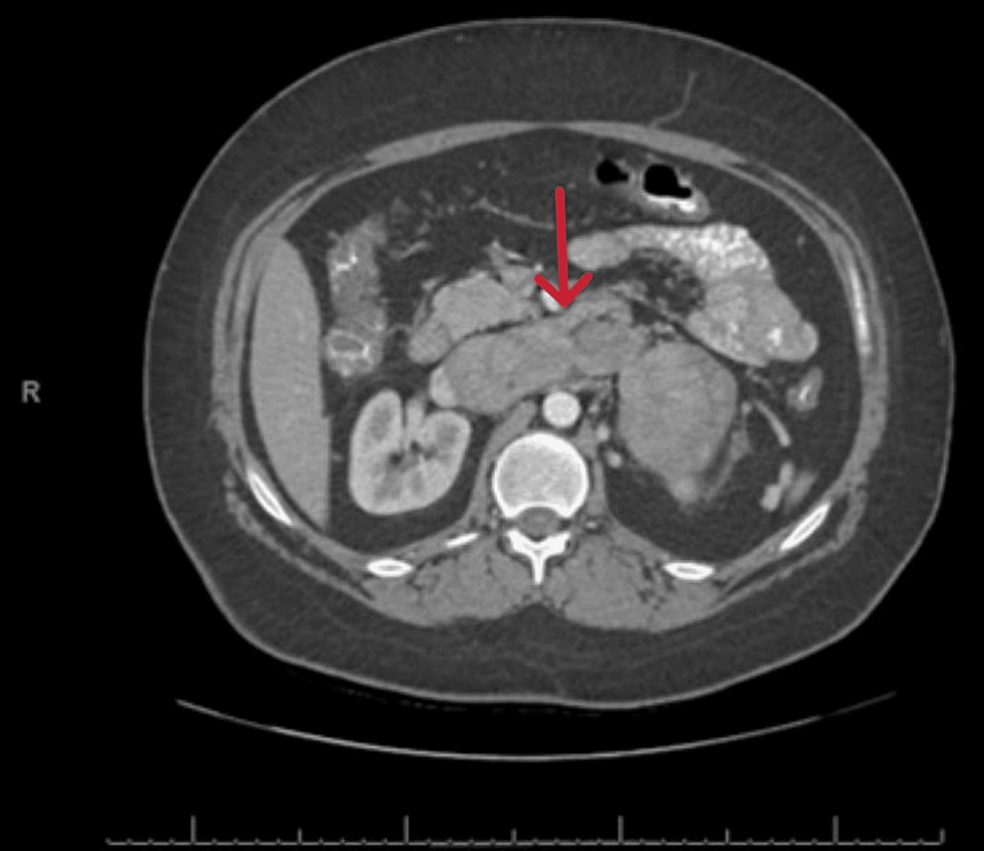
CT scan of the abdomen (axial view) with contrast showing the left adrenocortical carcinoma (red arrow) with extension into the inferior vena cava.
